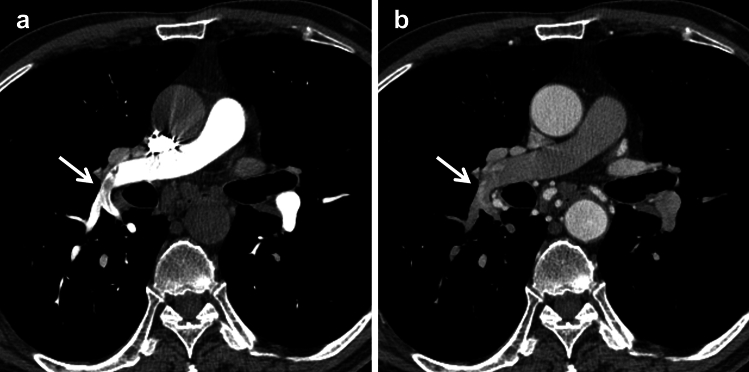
A 65-year-old man with CT image findings associated with systemic artery-to-pulmonary artery shunts (SPSs) a CT angiography for the pulmonary artery (CTA-P) shows filling defects in the main pulmonary artery to the inferior pulmonary artery (white arrow). b CT angiography for the systemic artery (CTA-S) shows enhancement in the pulmonary artery at the same part of the filling defects in CTA-P (white arrow). *CT* computed tomography
